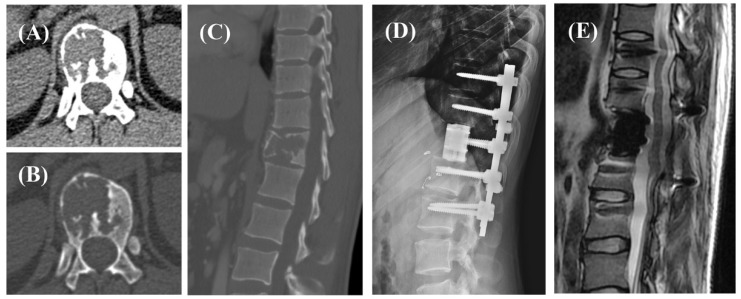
A 32-year-old male, CT images (A–C) showed a pathologic fracture of the T12 vertebra, managed with TES (D). Postoperative pathology showed low expression of RANKL without radiotherapy. At the 32-month follow-up, there was no evidence of recurrence (E).
